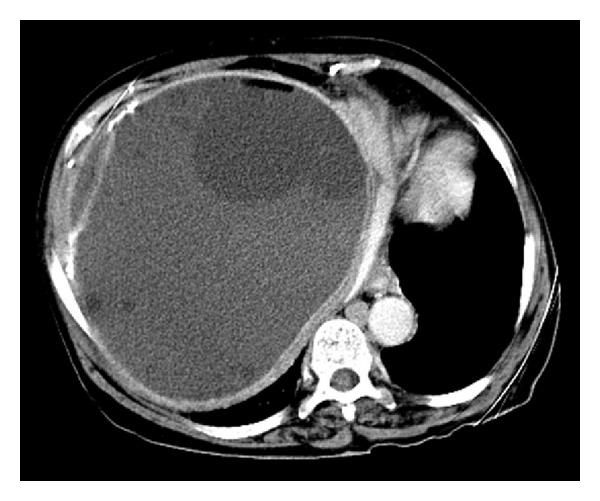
CT image of the thick-walled, complicated multicystic lesion and air density in it with a dimension of 19 cm × 19 cm × 20 cm.
